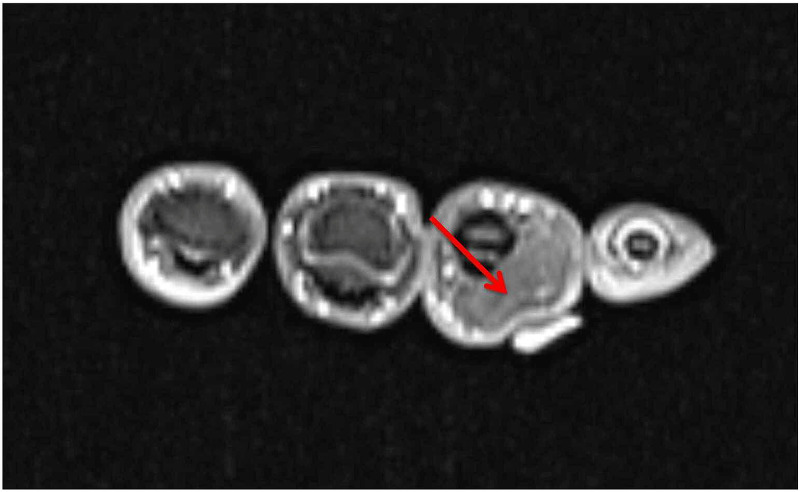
Axial MRI imaging of the right hand without gadolinium contrast enhancement; mass noted (arrow) MRI: magnetic resonance imaging
